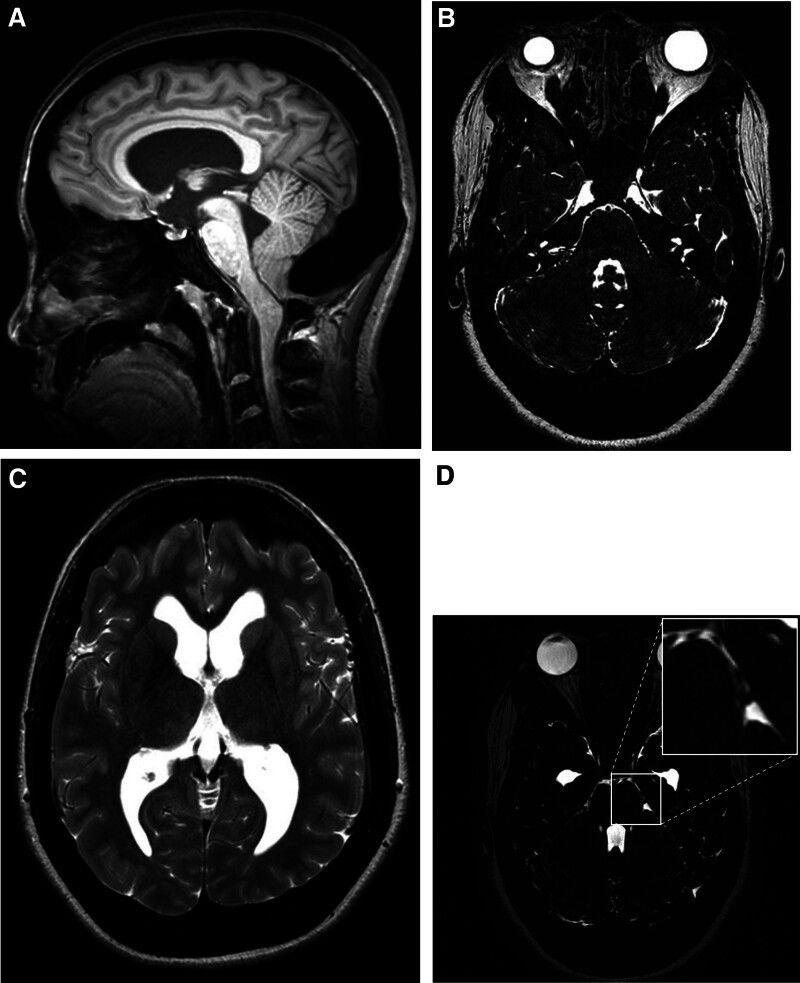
Magnetic resonance imaging prior to the first-stage decompressive surgery. (A) Sagittal T1-weighted image demonstrates posterior fossa crowding and brainstem compression caused by occipital bone overgrowth. (B) High-resolution T2-weighted image shows effacement of the cerebellopontine cisterns. (C) Axial T2-weighted image reveals mild ventricular enlargement, suggestive of obstructive hydrocephalus. (D) High-resolution T2-weighted image demonstrates limited visualization of the left trigeminal nerve and surrounding vessels due to severe cisternal crowding. The magnified inset highlights poorly defined neurovascular structures, raising suspicion for vascular compression of the trigeminal nerve.
